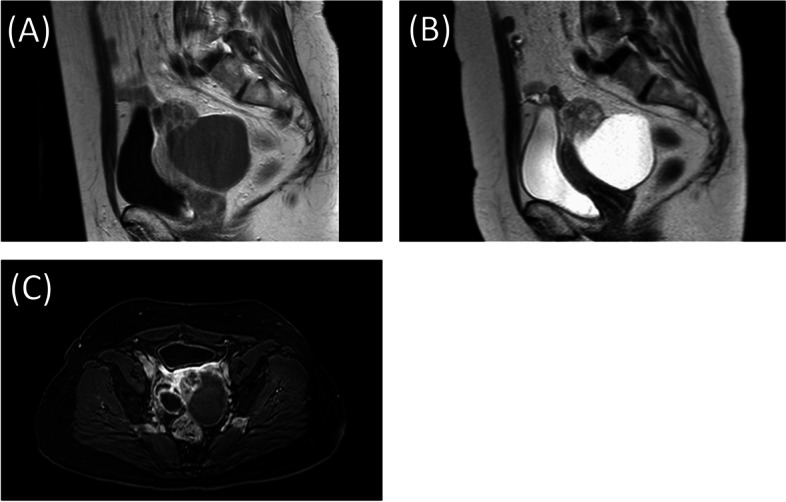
A T1-weighted MRI (B) T2-weighted MRI (C) Enhanced MRI. MRI with a 8.7 × 7.2 cm cystic and solid mass behind the uterus. A cystic lesion with a solid tumor was in the right adnexa. The solid lesion was well enhanced
